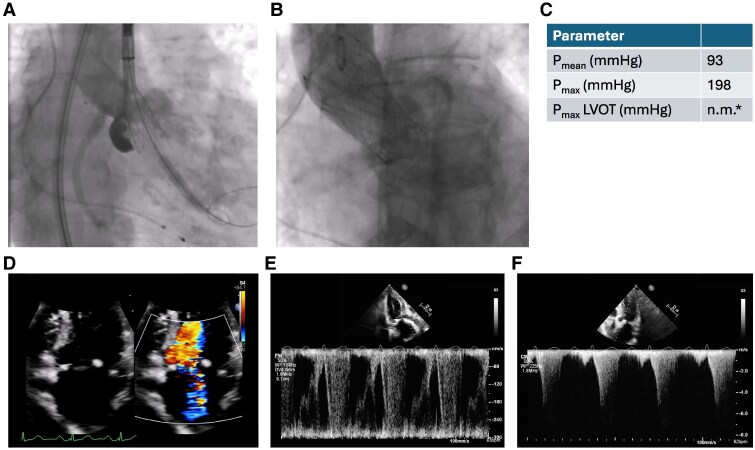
Transcatheter aortic valve implantation procedure and post-operative echocardiography. (*A*) Release of a self-expanding valve (Evolut Fx 26 mm, Medtronic, Minneapolis, MN, USA). (*B*) Transcatheter aortic valve implantation prosthesis after implantation without relevant aortic regurgitation. (*C*) Quantitative parameters in transthoracic echocardiography. P*_max_ in the left ventricular outflow tract was not measurable by PW-Doppler (see *P*_max_ by CW-Doppler). (*D*) Post-operative transthoracic echocardiography shows severe mitral regurgitation (*D*) and high left ventricular outflow tract gradients (*E* and *F*).
